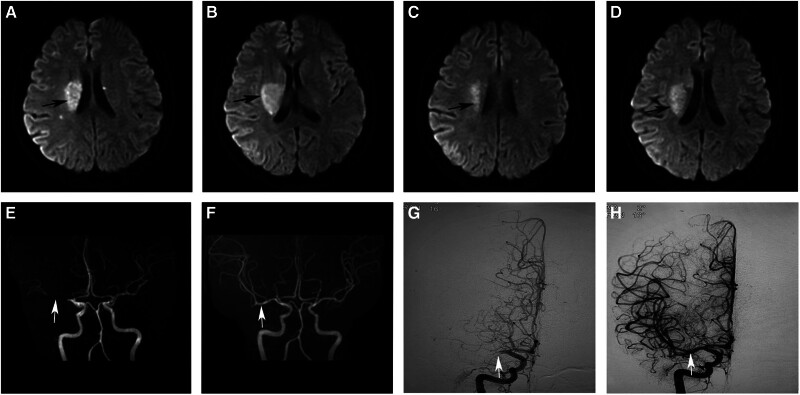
(A and B) Head MRI + DWI: multiple acute-phase cerebral infarctive changes in the right temporo-occipital insula, right basal ganglia, and bilateral radiocoronal-hemispheric centers. (C and D) Head MRI + DWI (reexamination): reduced signal intensity on T2WI and DWI sequences compared to previous. (E) Head MRA: the right middle cerebral artery occlusion. (F) Head MRA (reexamination): the right middle cerebral artery M1 section was visible, and the terminal branches were significantly more visible than before. (G) Cerebral angiogram (preoperative): the right middle cerebral artery M1 was occluded. (H) Cerebral angiography (postoperative): the right middle cerebral artery was patent. DWI = diffusion weighted imaging, MRA = magnetic resonance angiography, MRI = magnetic resonance imaging.
